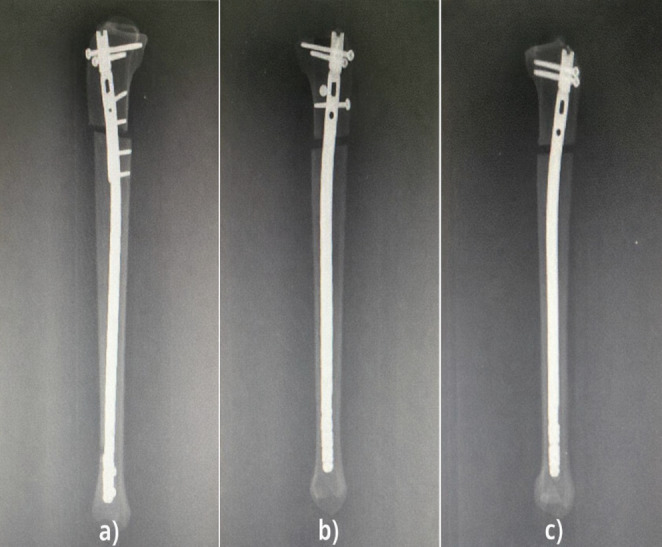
X-ray view (lateral radiography) of synthetic tibial bone models. a Intramedullary nail (IN) + plate. b Intramedullary nail (IN) + Poller screws. c Intramedullary nail (IN)
